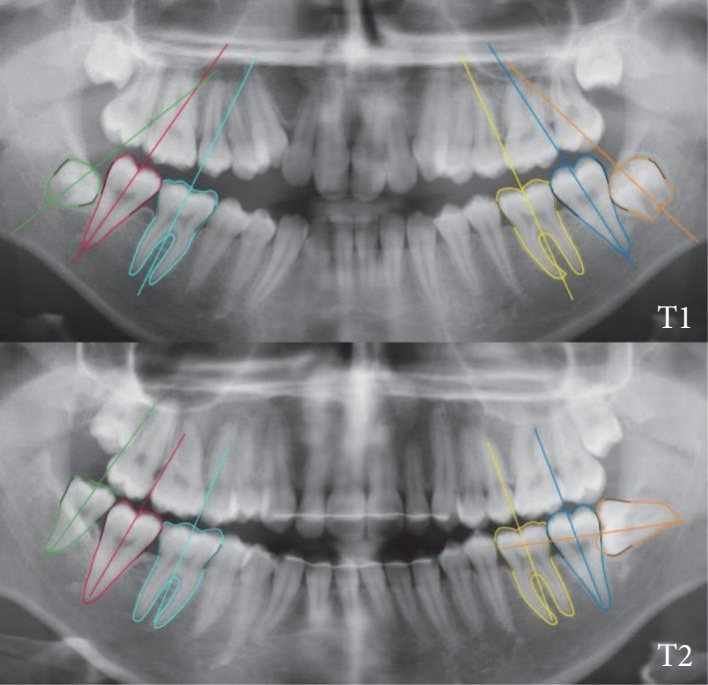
Consecutive panoramic radiographs of a patient with unilateral fainting wisdom tooth: (T1) upright wisdom tooth germs on both sides and (T2) vertically erupted wisdom tooth on right side and fainting wisdom tooth on left side.
